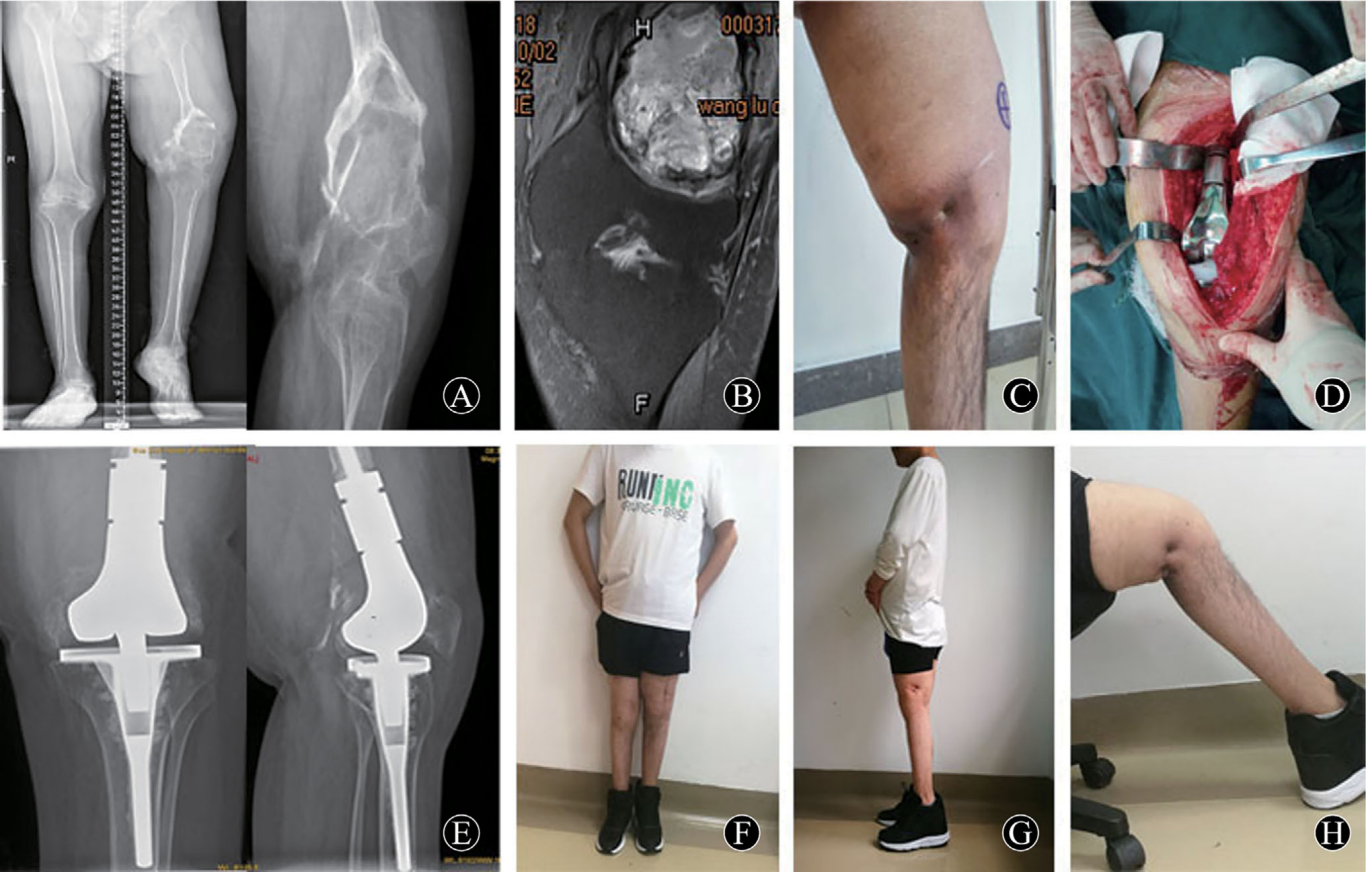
A 23‐year‐old man (patient 9) who had a 3‐year history of left distal femoral pain and progressive swelling. His diagnosis was a hemophilic pseudotumor with severe hemophilic knee arthritis. (A) Preoperative X‐ray for double lower‐limb full‐length, left lower limb was 12.0 cm shorter than the right lower limb. Preoperative lateral X‐ray showed expansile osteolytic destruction of the distal femur extending to the articular surface. Multiple bone septa were present in the lesion area. (B) Preoperative MRI T2 image in the coronal plane, heterogeneous signal intensity was present. The margin of between the lesion and adjacent area tissue was clear. (C) Preoperative physical examination of left lower limb showed distal femoral swelling and two fistulas. (D) Intraoperative photograph for knee prosthesis. (E) Postoperative photograph taken 24 months after surgery, the implants were stable. (F) Photograph of standing position taken 24 months after surgery. The patient was satisfied with wearing orthopaedic shoes. (G) Photograph of lateral standing position taken 24 months after surgery, the knee fully extended to 0°. (H) The left knee could flex to 40°. The fistulas had completely disappeared.
